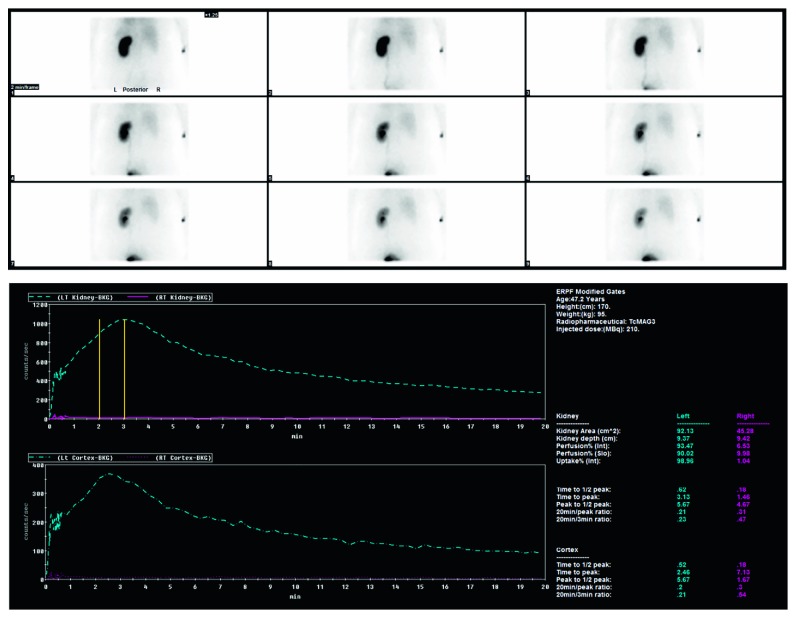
MAG3 scintigraphy showing complete loss of function of the right kidney.
